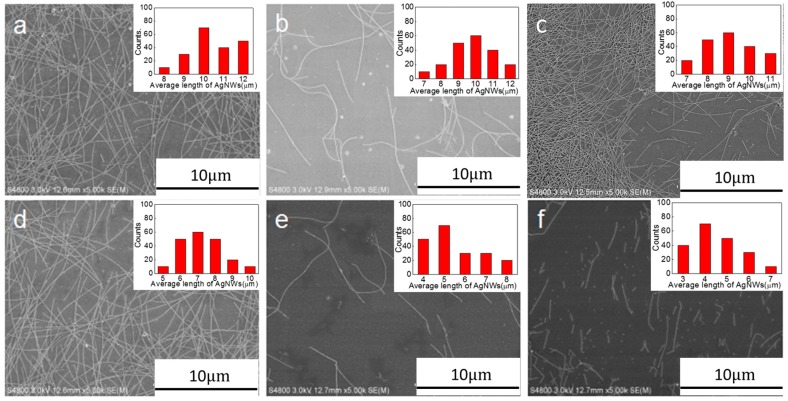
SEM images of AgNWs subjected to sonication-induced scission at an ultrasonic power of 300 W for (a) 0.5, (b) 1.0, (c) 1.5, (d) 2.0, (e) 2.5, and (f) 3 h. Insets show the corresponding AgNWs length distributions.
